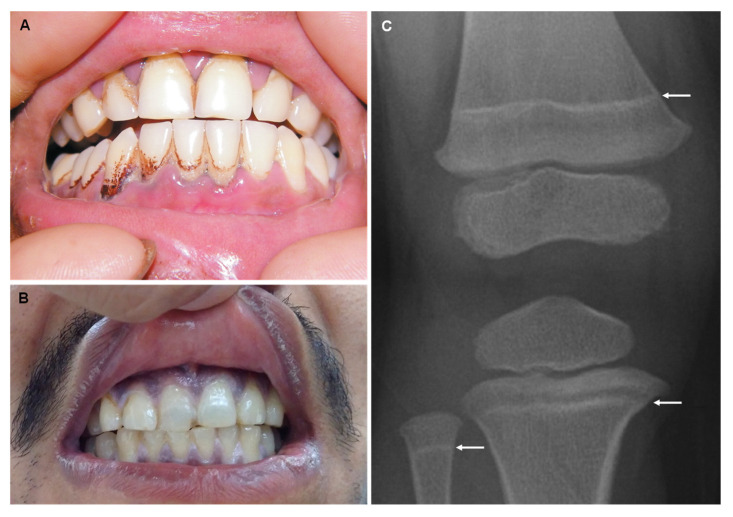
Characteristic Burton’s lines are highlighted by the deposits (A) and blue-purplish discoloration of the gums (B). X-ray with arrows showing “lead lines” (C). Reprinted from (A)—[94], (B)—[95], (C)—[96].
